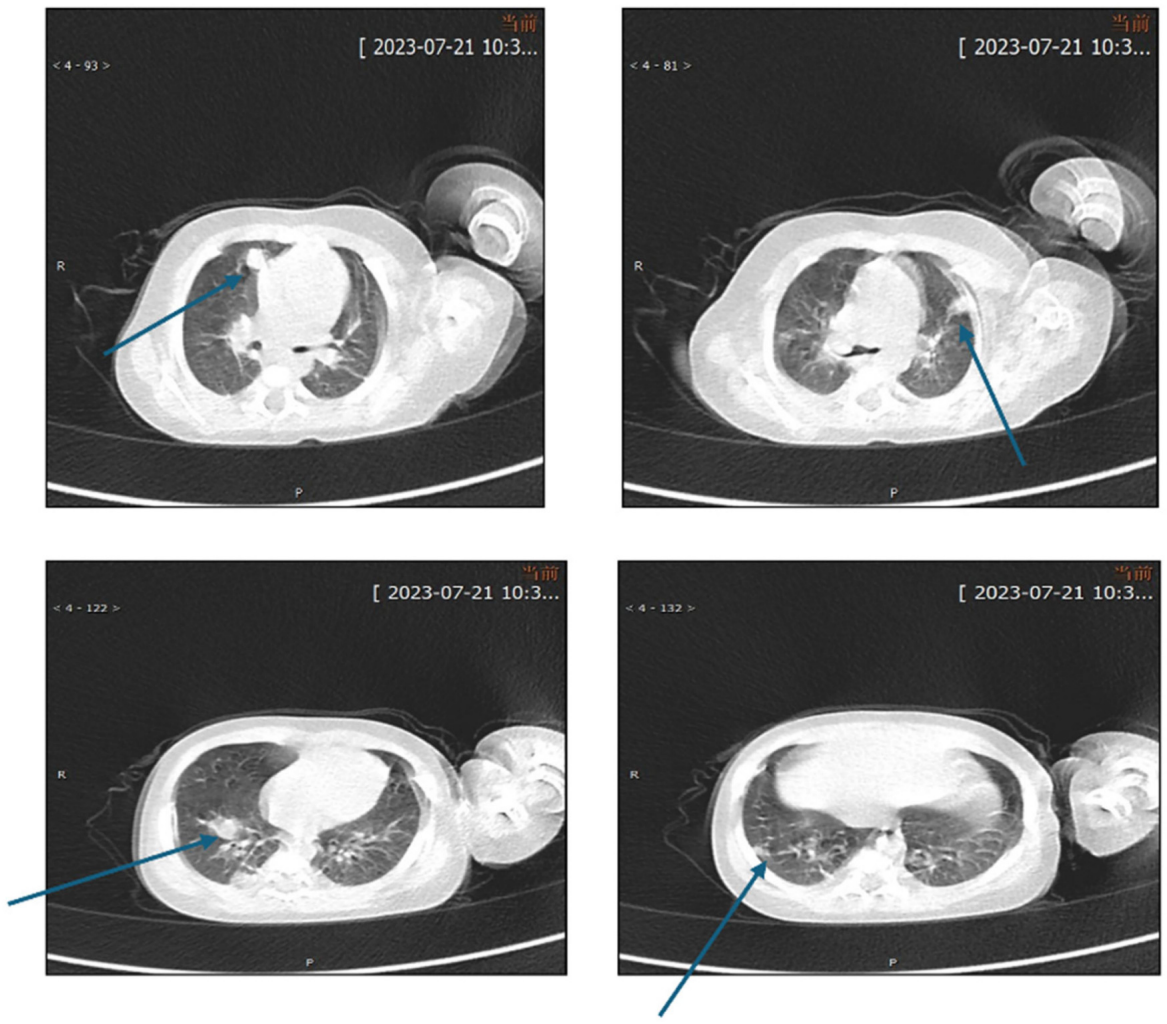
Chest CT obtained after 1 week of treatment showed partial radiological improvement of pulmonary lesions.
